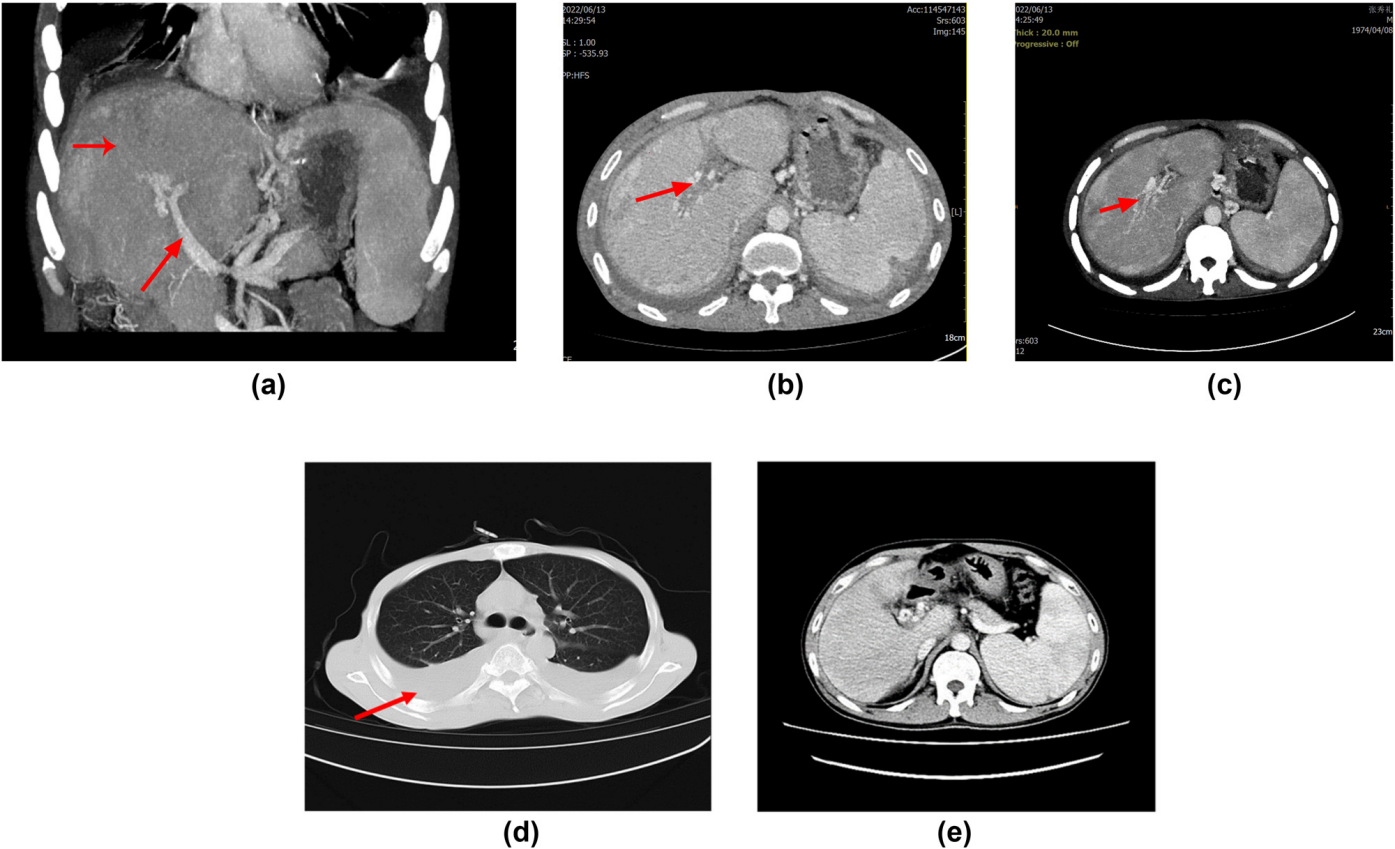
Imaging examination: (a) During reexamination in 2022: Significant worsening of main portal vein stenosis (indicated by the red arrow) with partial intrahepatic branches essentially having disappeared. In the portal venous phase of CT, significantly lower enhancement in most of the liver than in the spleen (indicated by the red arrow), significantly more aggravated portal hypertension, significantly thicker varices in the esophagus and stomach fundus, and a thicker splenorenal venous shunt. (b) The portal vein area shows thrombosis (indicated by the red arrow). (c) Spongy changes in the portal vein area (indicated by the red arrow). (d and e) Bilateral pleural effusion with no obvious signs of ascites.
